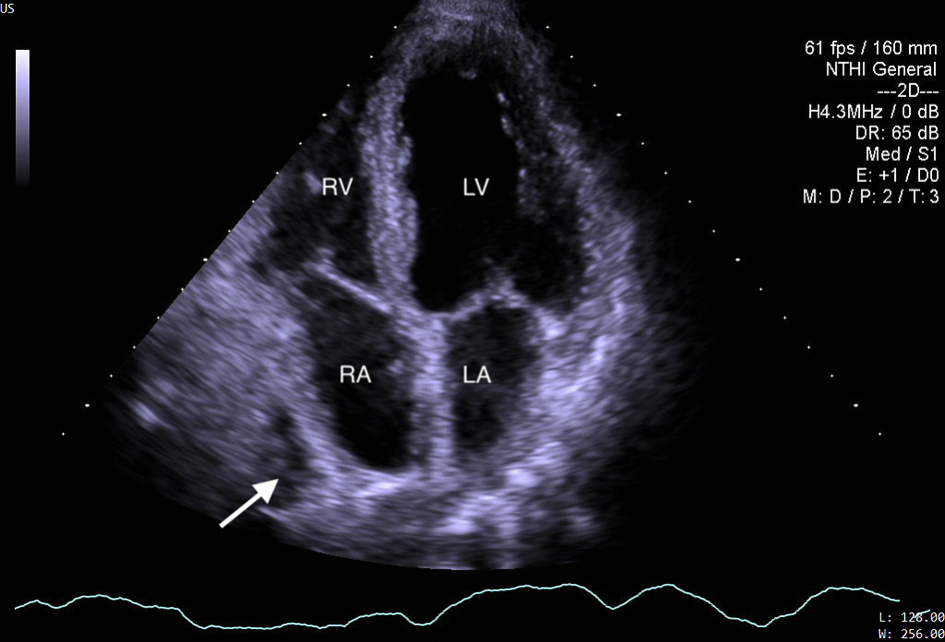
Recovery of right atrial function on postsurgical transthoracic echocardiography. Small hepatic cyst after laparoscopic deroofing (*arrow*).
